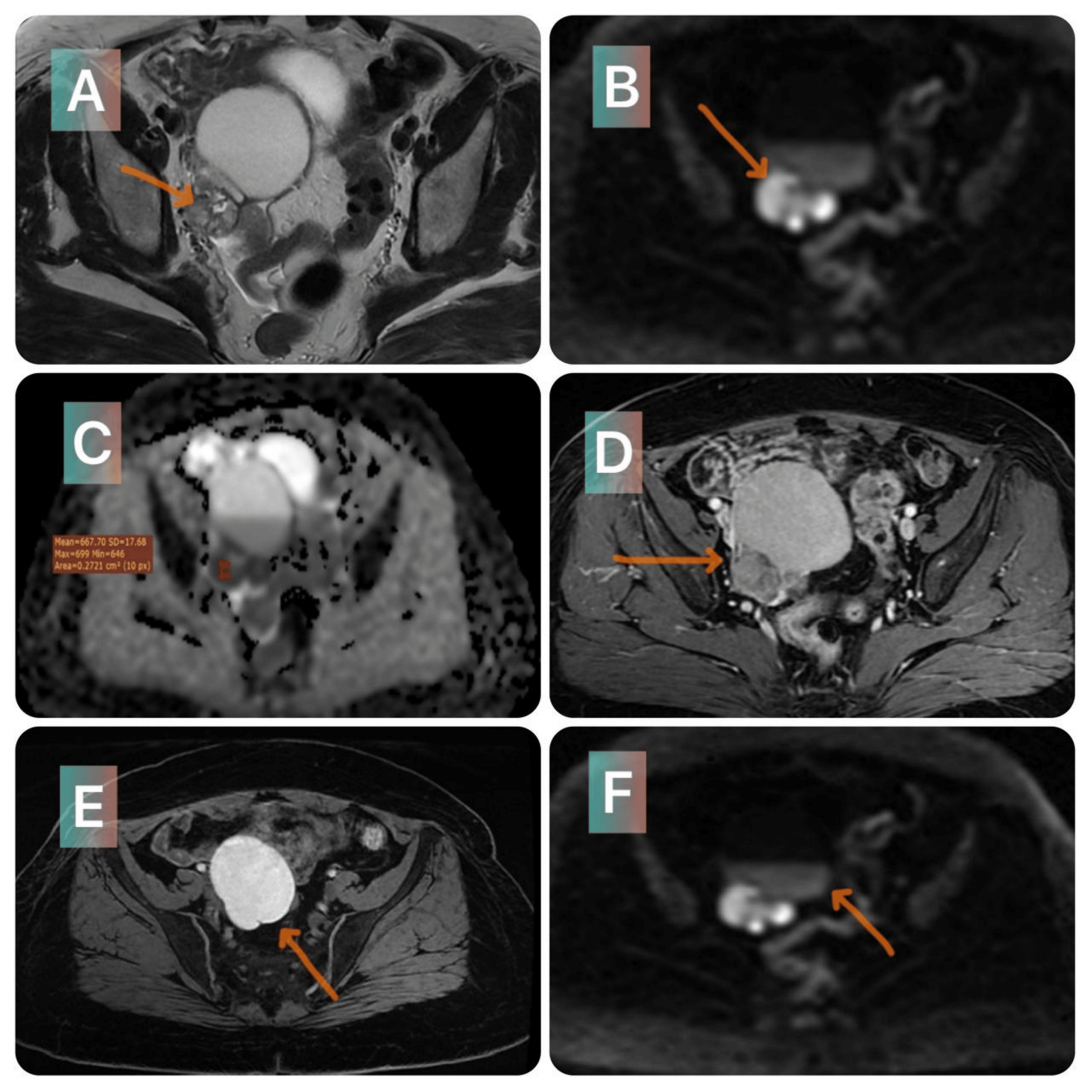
Pelvic MRI. MRI in axial T2 sequence, without fat saturation before and after gadolinium injection + diffusion sequences with apparent diffusion coefficient (ADC) mapping: Solidocystic mass, with tissue portion in intermediate T2 signal (A), diffusion hyper signal with drop in ADC (B and C), enhanced after gadolinium injection (D). T1 fat-saturated and diffusion sequence: Multiloculated fluid portion, with two thin-walled cubicles, with hemorrhagic content in hypersignal T1 (E), containing a liquid-liquid level visible at the sequence diffusion (F).
